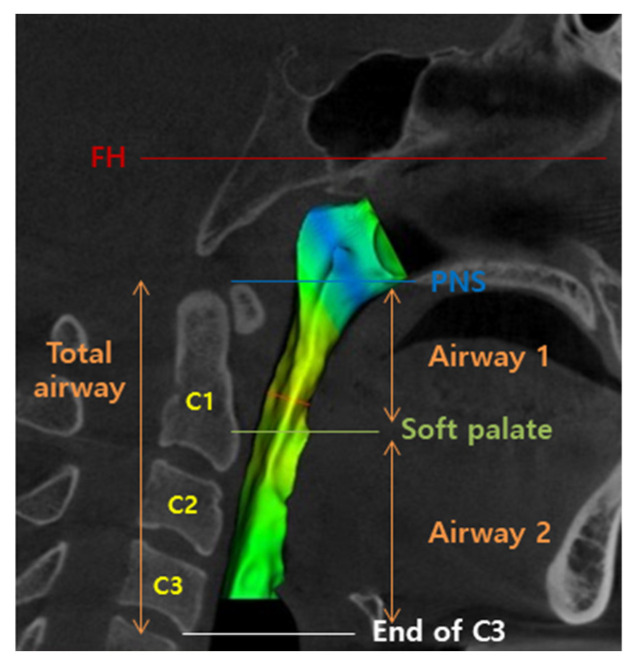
Demonstration of pharyngeal airway section with the volumetric analysis on the CT.
